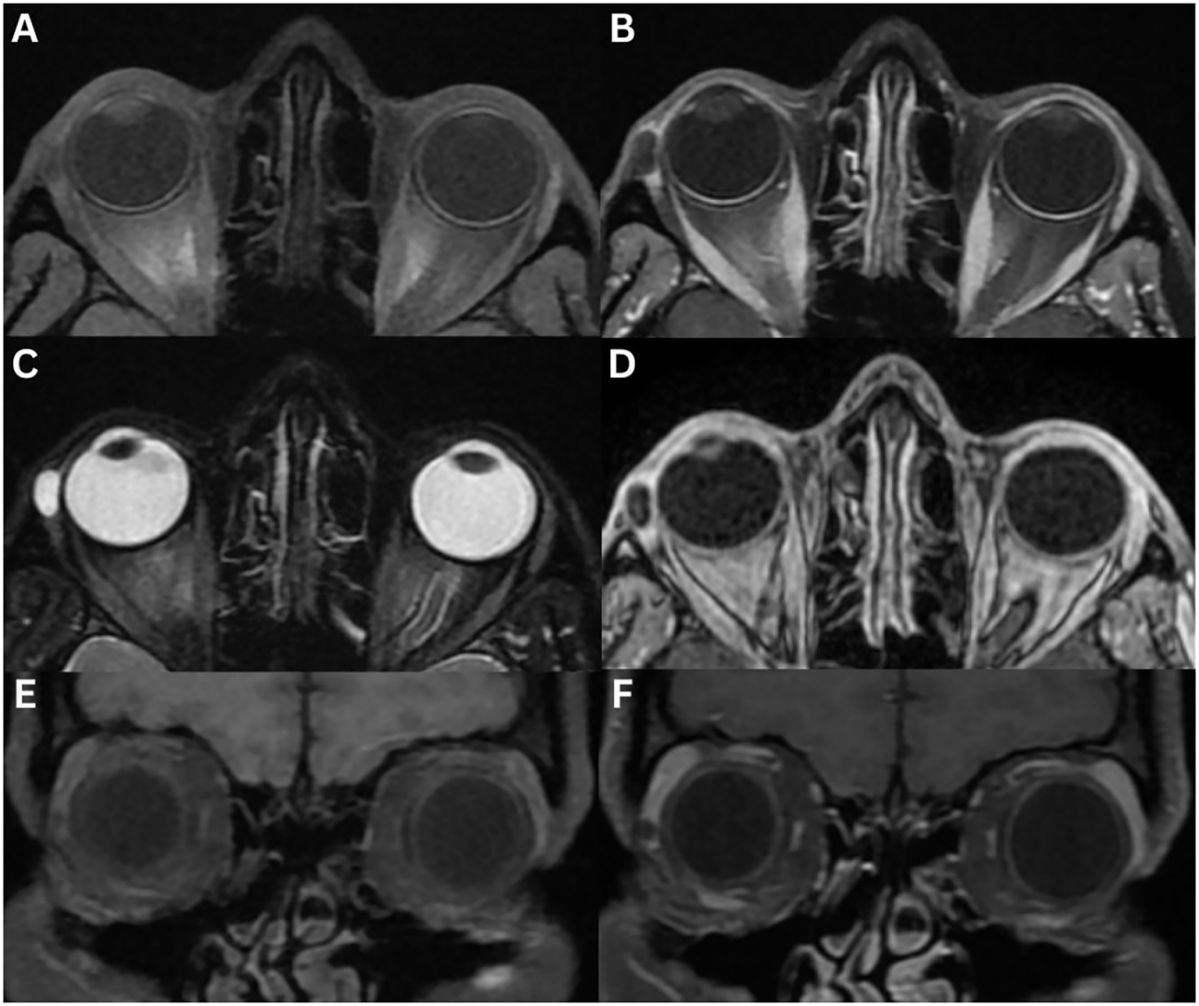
Dacryops of the right lacrimal gland. MRI T1-weighted axial fat-saturated (A) and axial fat-saturated contrast-enhanced (B), T2-weighted axial fat-suppressed (C), axial 3D contrast-enhanced (D), and T1-weighted coronal fat-suppressed (E) and coronal fat-suppressed contrast-enhanced (F) sequences demonstrate a right superficial palpebral lobe cystic lesion. There is no contrast enhancement and no mass effect on adjacent structures.
